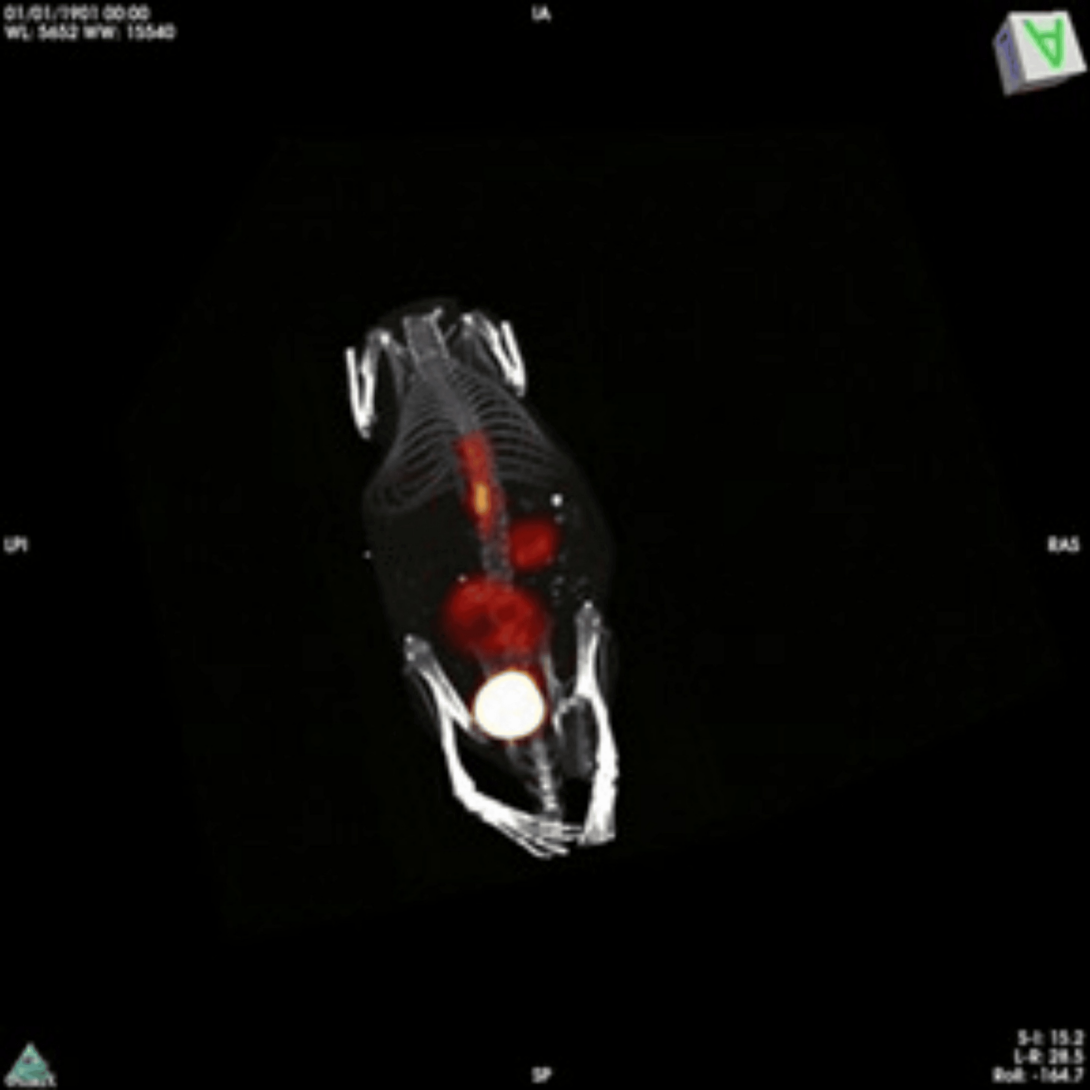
PET-CT performed in the model three weeks post-surgery, showing evidence of 18-FDG uptake as the contrast agent in the lumbar vertebrae at the site where the tumor cells were inoculated, along with areas of external uptake, which are attributed to a probable infectious process.
